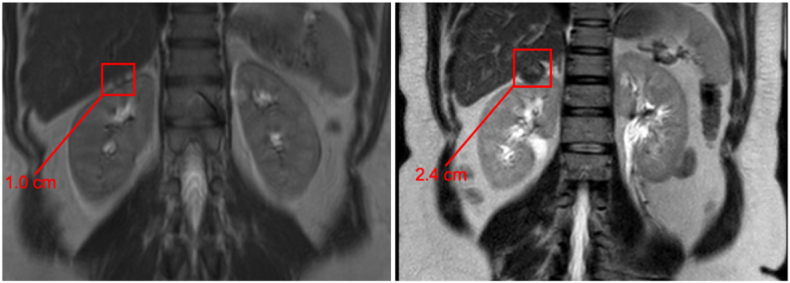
T2 weighted coronal MRI images of right renal mass on presentation (right) and six months prior (left).
